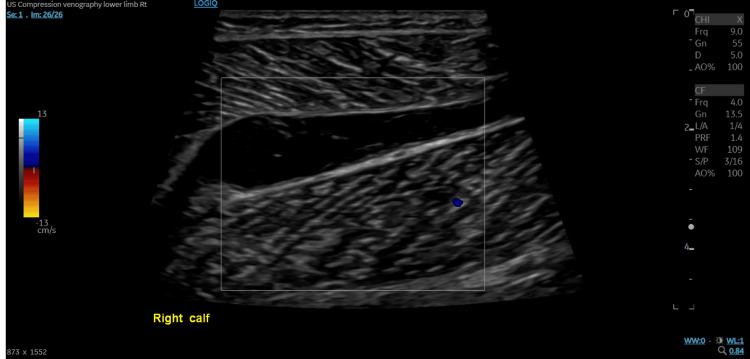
Ultrasound compression venography of the right calf demonstrating an avascular, cystic area with features suggestive of hemorrhage
